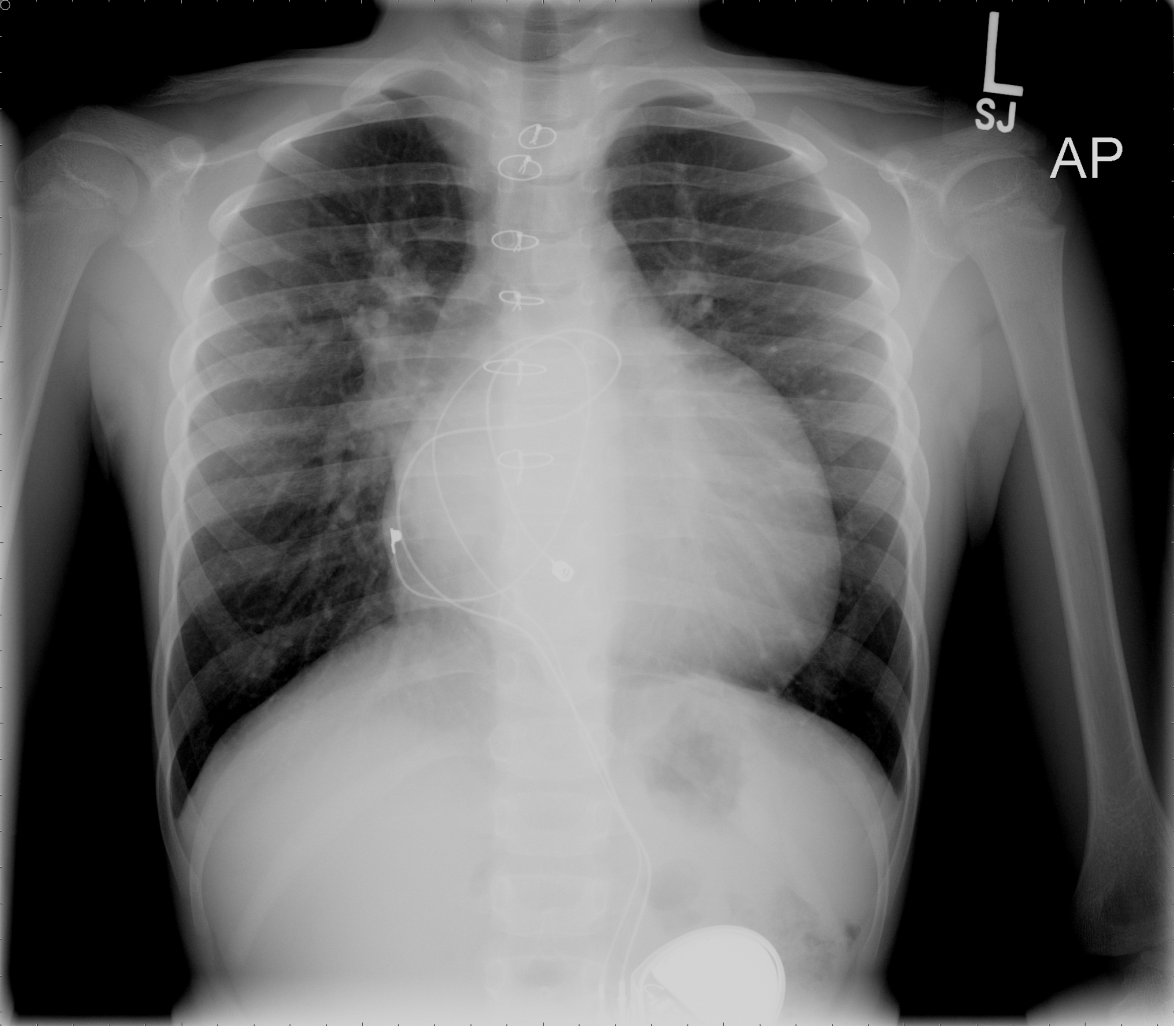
The upper part of cardiac silhouette as seen in the chest radiograph appears abnormally straight because of the loss of the normal arterial relationships.
